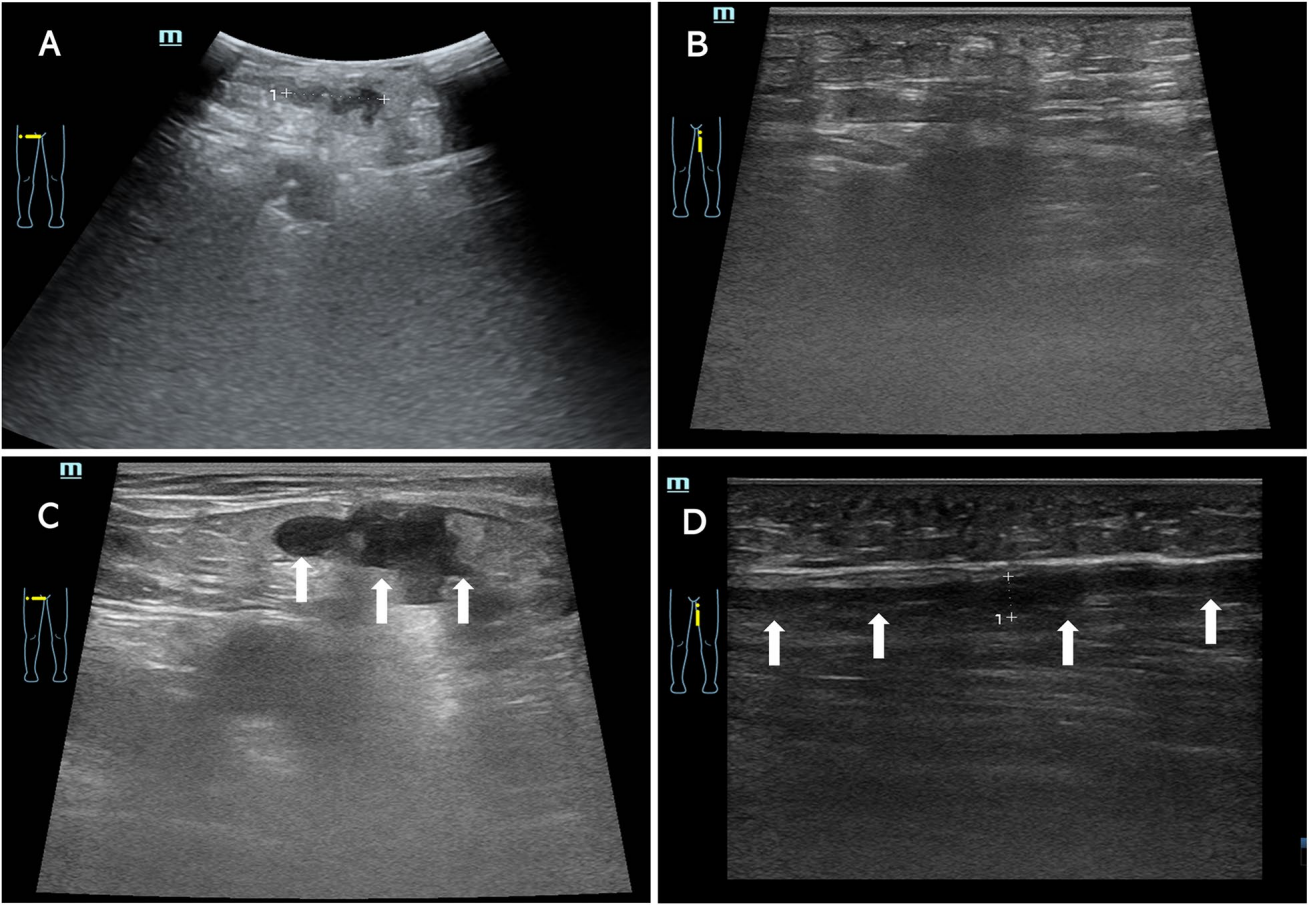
Lower extremity ultrasound images of the HIT patient. The initial ultrasound examination only showed a localized hematoma in the right thigh (A) with no significant hemorrhage sign in the left thigh (B). The second ultrasound examination indicated the enlarged hematoma in the right thigh (C) and new developed subcutaneous hemorrhage in the left thigh (D)
